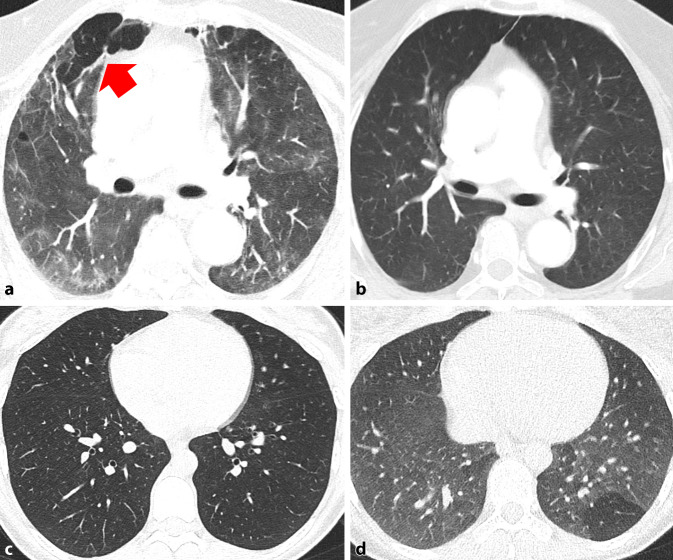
Aufgrund des Ausmaßes der Lungenbeteiligung, begleitenden Pathologien und der mechanischen Beatmung bilden die COVID-19-ARDS-Überlebenden eine besondere Gruppe von Patienten. Drei Monate nach COVID-19 zeigten die CT-Scans bei 70–80 % dieser Patienten weiterhin Auffälligkeiten. Insgesamt wiesen diese Patienten ein breites Spektrum an Veränderungen wie Milchglas und retikulären Verdichtungen allein oder in Kombination, Konsolidierungen, interlobulären septalen Verdichtungen, Bronchiektasien und Architekturstörungen auf ([2, 11, 14], Abb. 3). Das Ausmaß der persistierenden radiologischen Läsionen korrelierte mit der Anzahl der Tage auf der Intensivstation, während die mechanische Beatmung mit Zeichen, die auf eine Fibrose hinweisen (Kombination von Retikulationen und Traktionsbronchiektasien) assoziiert war [11]. Der Begriff „Traktionsbronchiektasie“, der auf irreversible fibrotische Veränderungen hindeutet, sollte in diesem Zusammenhang jedoch mit Vorsicht verwendet werden, da eine bronchiale Dilatation und Distorsion in Bereichen von Konsolidierungen oder Milchglasverdichtungen im längerfristigen Verlauf reversibel sein kann (Abb. 3, [22]). Daher sollten diese Veränderungen als „fibroseähnliche Veränderungen“ bezeichnet werden, die wahrscheinlich Residuen einer (abgelaufenen) organisierenden Pneumonie widerspiegeln [22]. Ein Honeycombing, welches das definitive CT-Merkmal einer Lungenfibrose darstellt, wurde nur in anekdotischen Fallberichten beschrieben. Dieses kann möglicherweise mit einer vorbestehenden fibrosierenden Lungenerkrankung in Verbindung gebracht werden [22].
